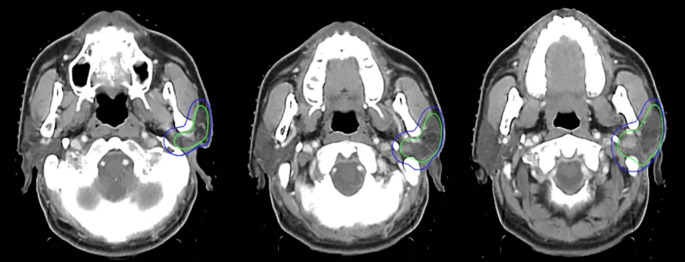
Axial contrast-enhanced planning CT (blue line: PTV; green line: CTV).
